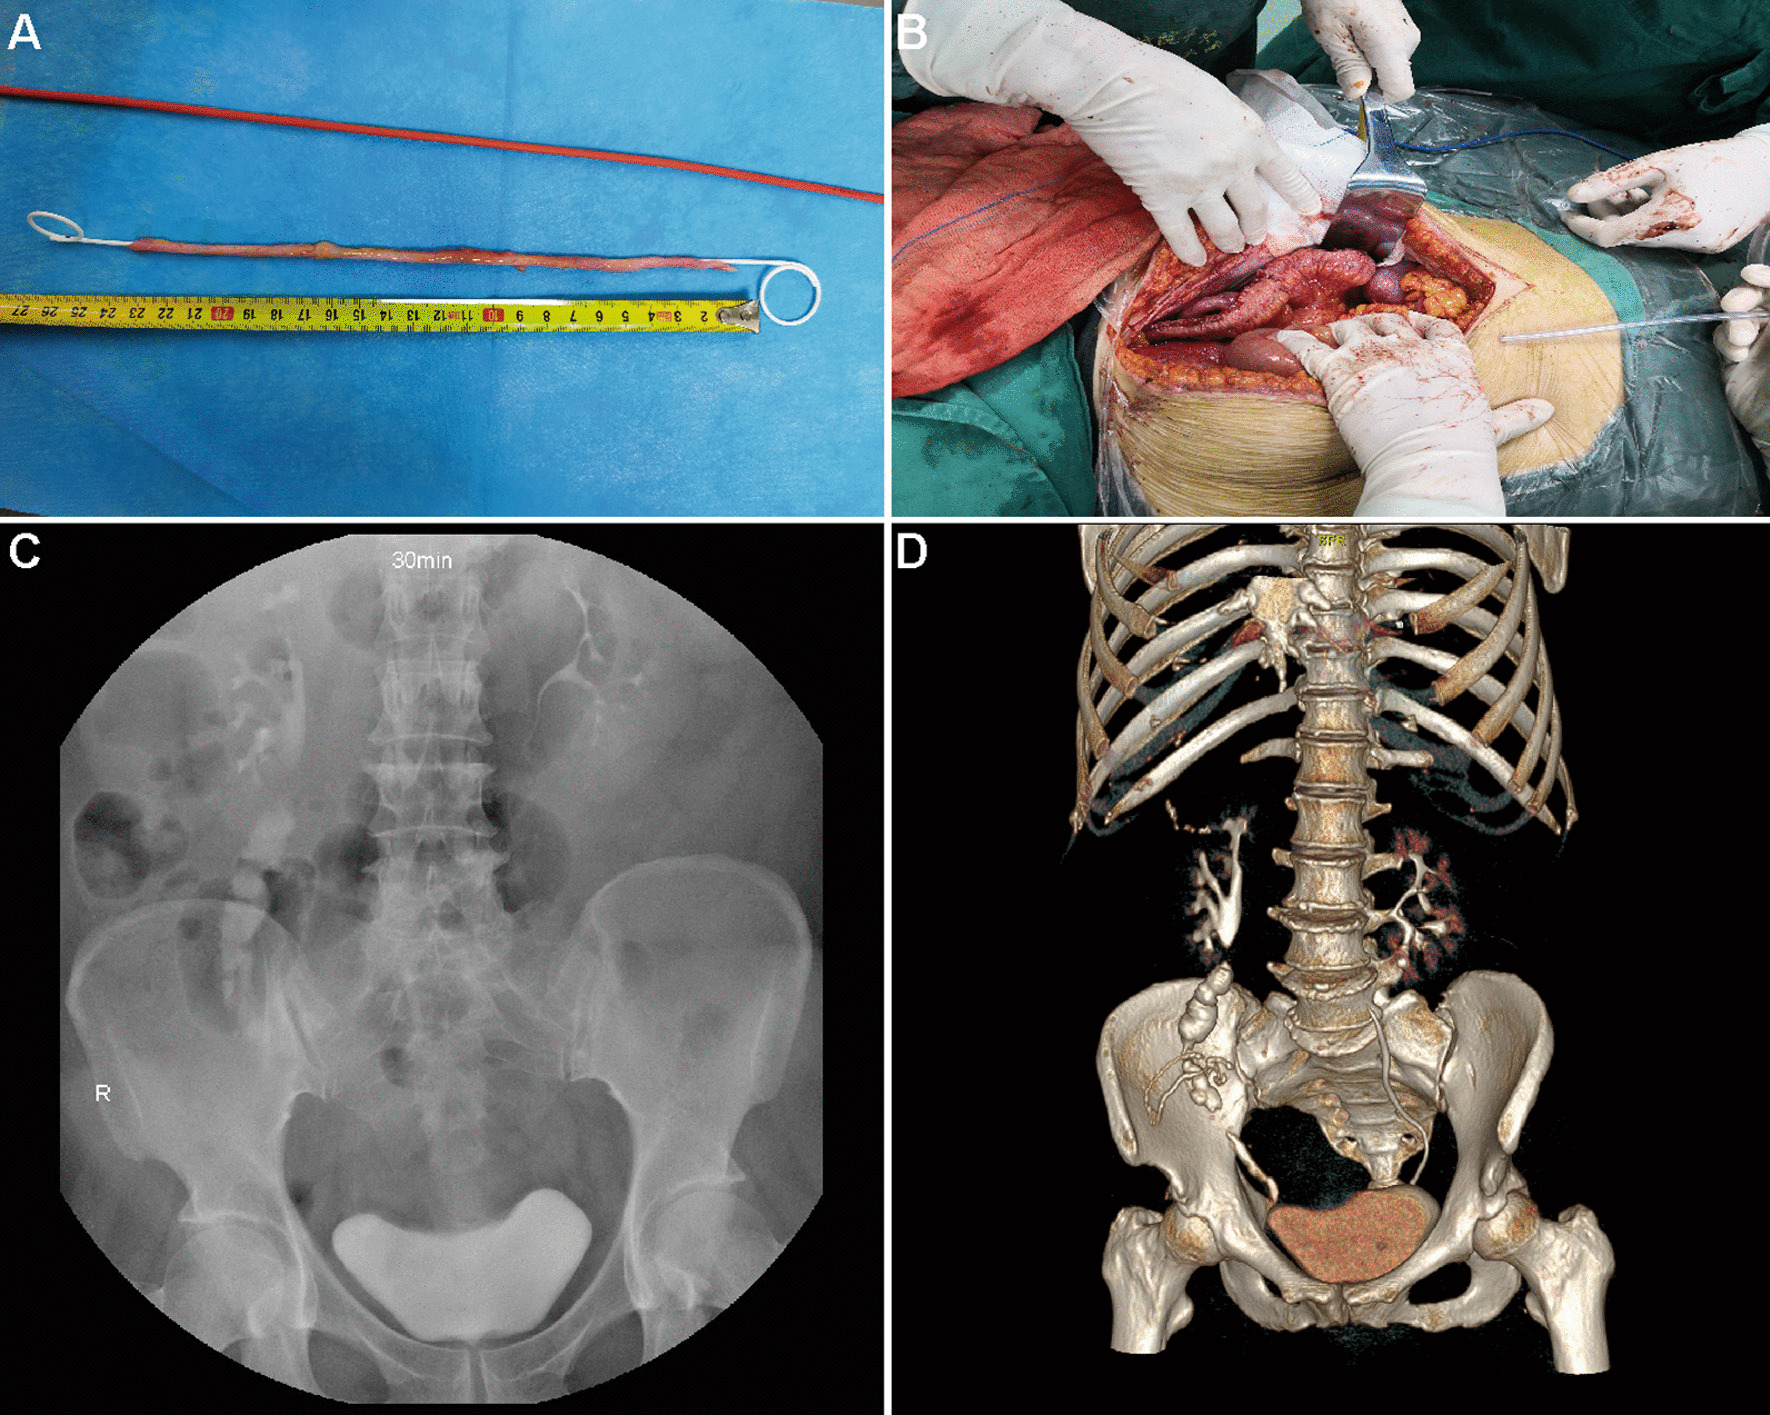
Images of patient 2. A Avulsed panureter approximately 23 cm in length. B Bridging the ureter by retubularized TDI. C Intravenous urogram in months 9 post-surgery. D CT urogram in month 27 post-surgery
